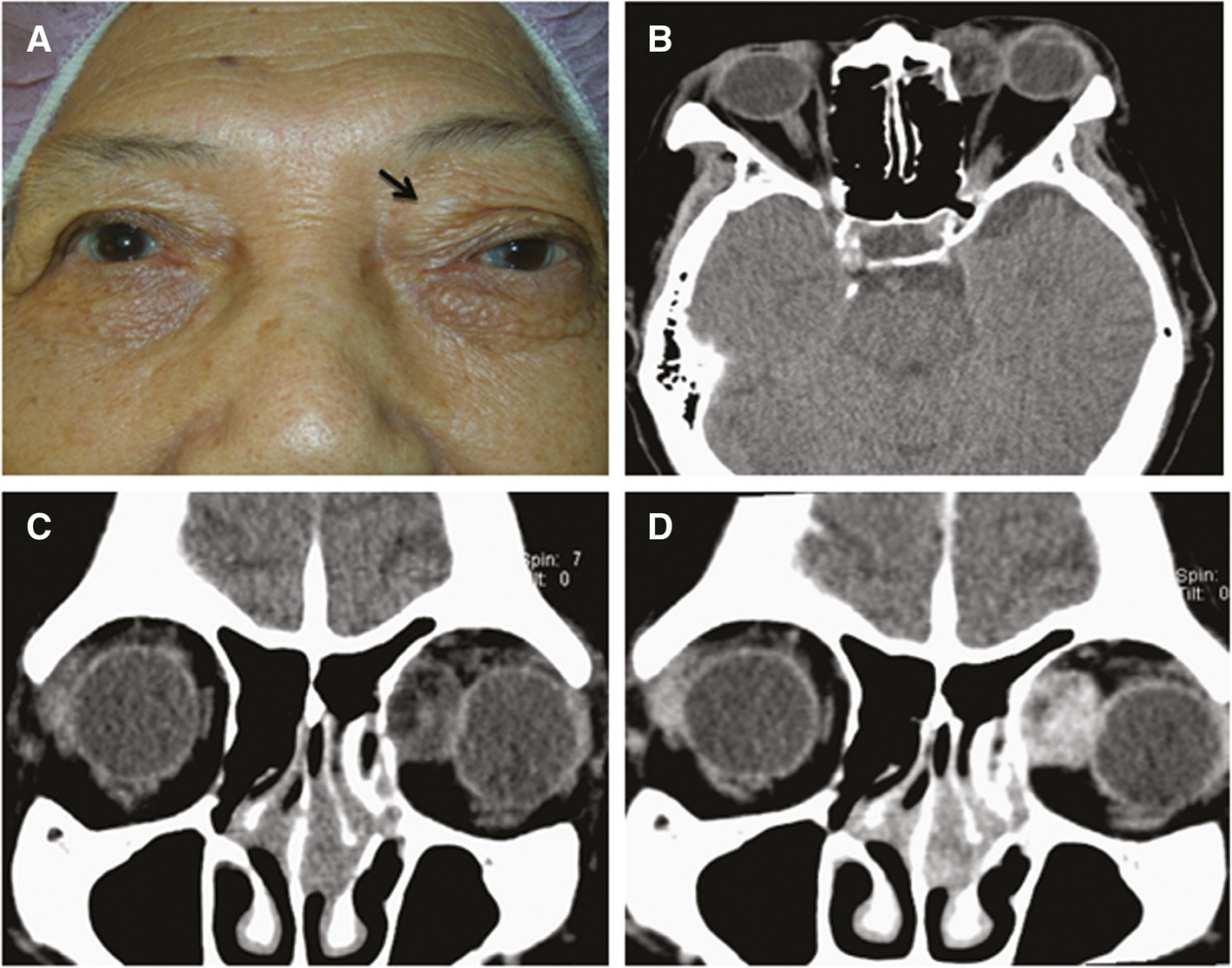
Preoperative photograph shows a palpable mass on left superonasal orbit (a, *arrow*). Axial (b) and coronal (c) planes of computed tomographic scan show a circumscribed soft tissue mass on the left superonasal orbit with heterogenic density containing areas of fat attenuation. The mass demonstrates heterogenous enhancement (d)
